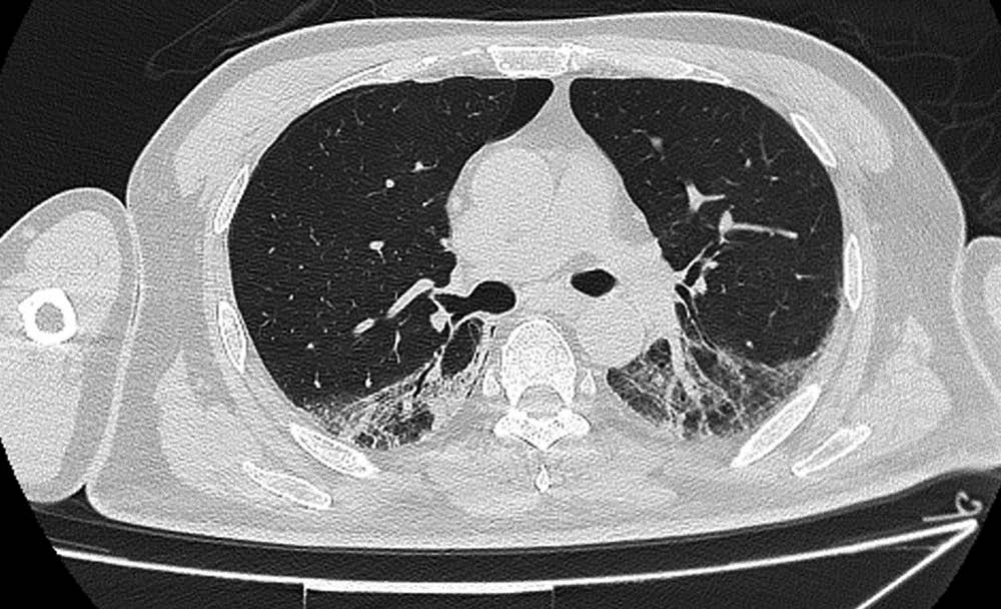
Chest high-resolution computed tomography on July 7, 2024 showed a few ground glass shadows in both lungs.
